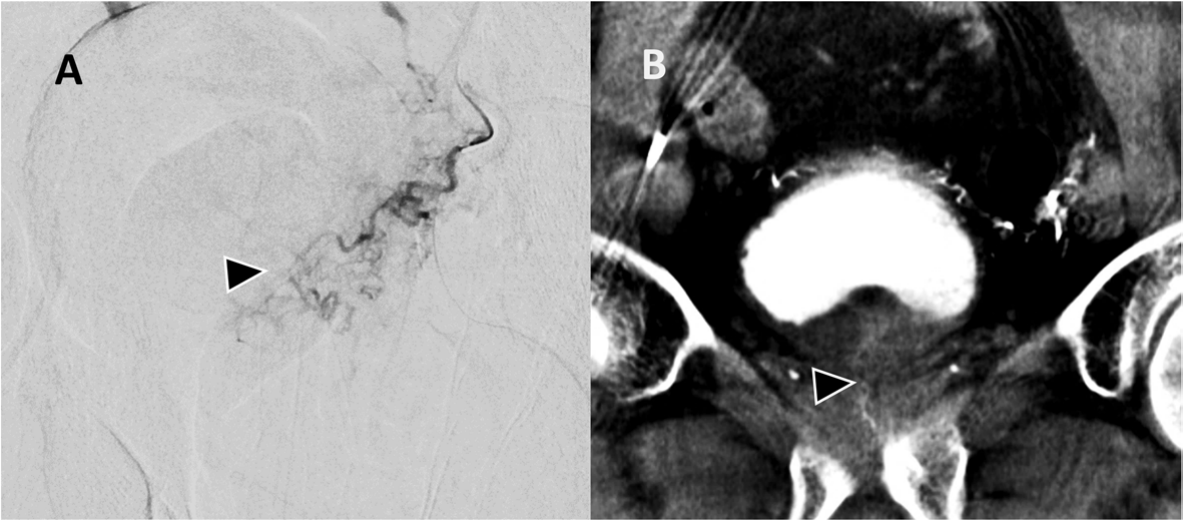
A Selective DSA of the left prostatic artery (black arrowhead) with a typical course of prostatic artery but the perfusion blush is not convincingly depicting perfusion of the prostate. This was not a very enlarged gland (70 mL). B) CBCT confirmed perfusion of the left lobe of the prostate (black arrowhead). Small vesical branches seen at the dome of the bladder are due to reflux from power injection during CBCT and were not visualized or included during embolization
